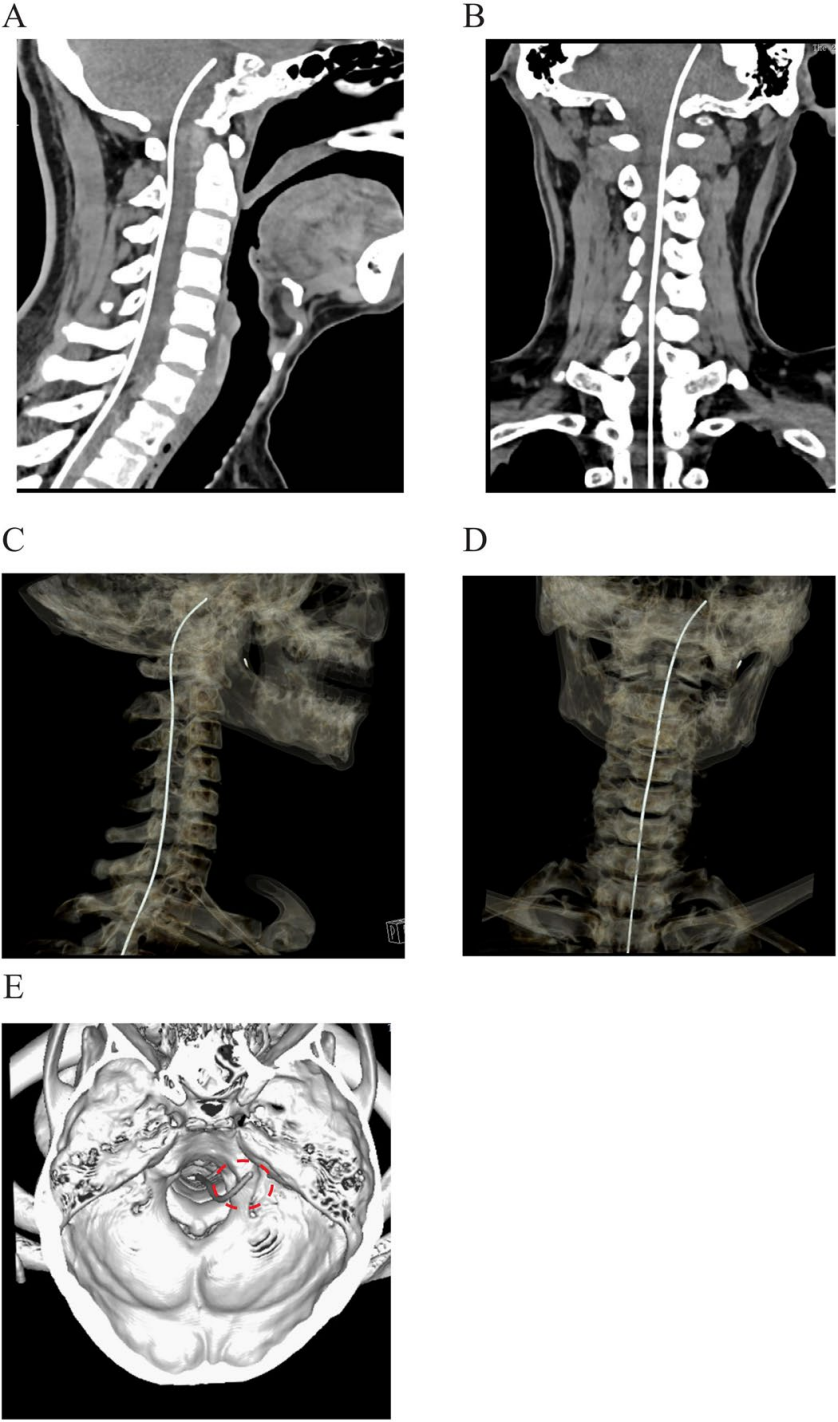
Postoperative imaging of catheter placement with computed tomography. a, b: Sagittal and coronal plane of scanning demonstrated that the tip of intrathecal catheter was located at the level of posterior clinoid process. c, d: Three-dimensional reconstruction of catheter placement by computed tomography. e: Reconstruction of the skull base to reveal the location of the catheter (circled by the red dash line).
